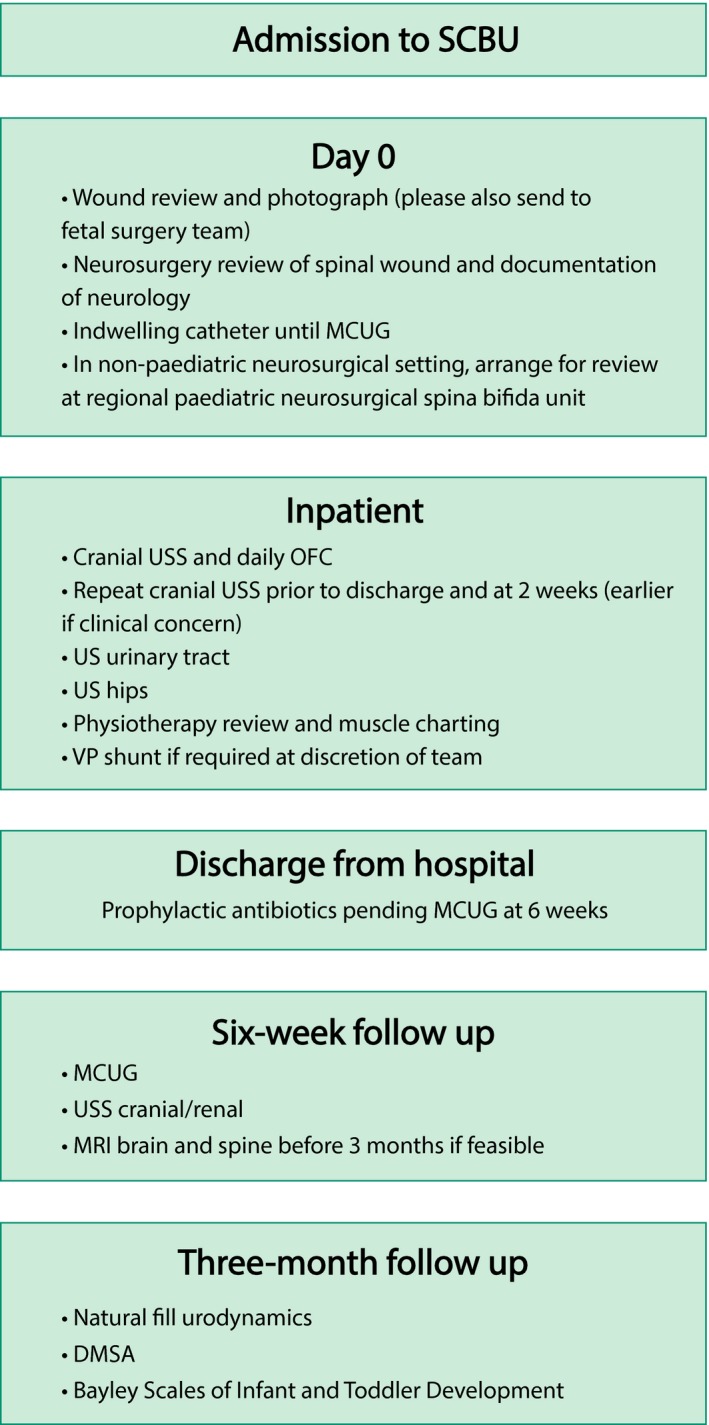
Neonatal protocol following open fetal surgery for spina bifida. DMSA = dimercaptosuccinic acid radionuclide scan; MCUG = micturating cystourethrogram; MRI = magnetic resonance imaging; OFC = occipito‐frontal circumference; SCBU = special care baby unit; USS = ultrasound scan; VP = ventriculoperitoneal
